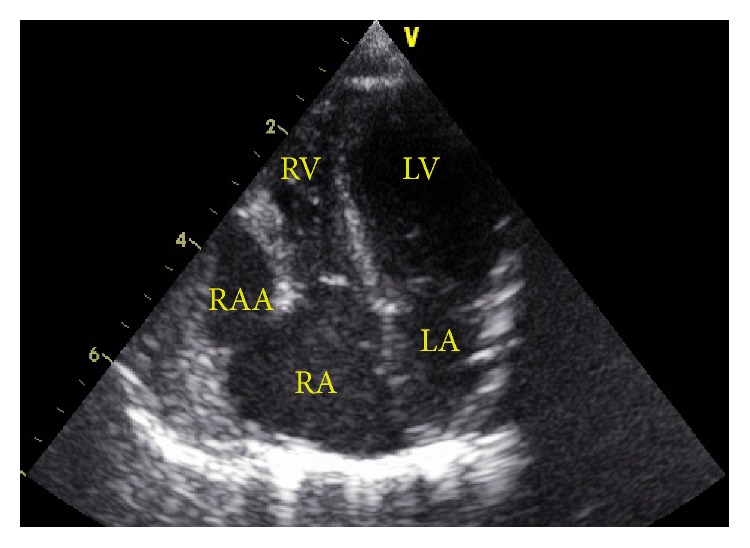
Transthoracic echocardiography revealed an enlargement of the aneurysm on anterior and right of the RA (RAA) (RAA: right atrial appendage; RA: atrium; RV: right ventricle; LA: left atrium; LV: left ventricle.).
